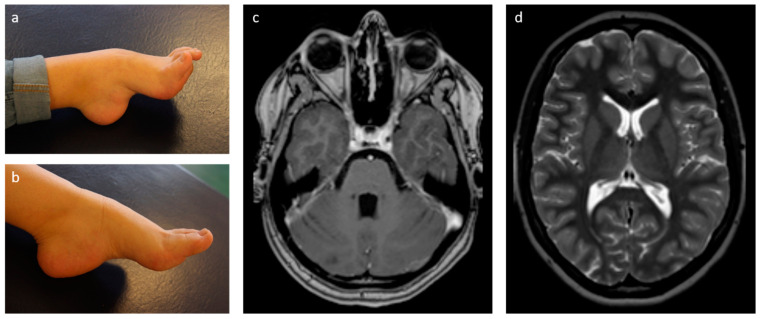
Pes cavus deformity in daughter (a) and mother (b) with a pathogenic variant in *SAMD9L*. (c) Cerebral MRI of the daughter at the age of 15 years showed some minimally accentuated cerebellar fissures on axial T1 weighted images. (d) The supratentorial brain was unremarkable according to age as shown on the axial T2 weighted images.
